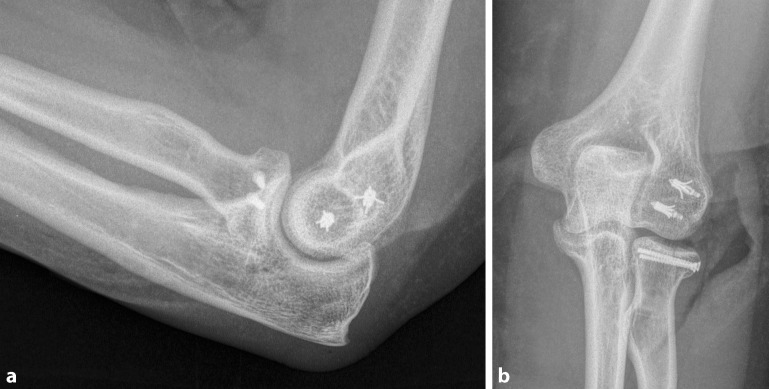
a Lateral and b anterior–posterior radiographs of the injured elbow. Capitellar fracture (type III) with associated Mason-II fracture of the radial head and tear of the lateral collateral ligament after operative treatment. The capitellar fracture was treated with bioabsorbable pins, torn ligaments were refixated using Mitek anchors, and the radial head fracture was osteosynthesized with two small-fragment screws
